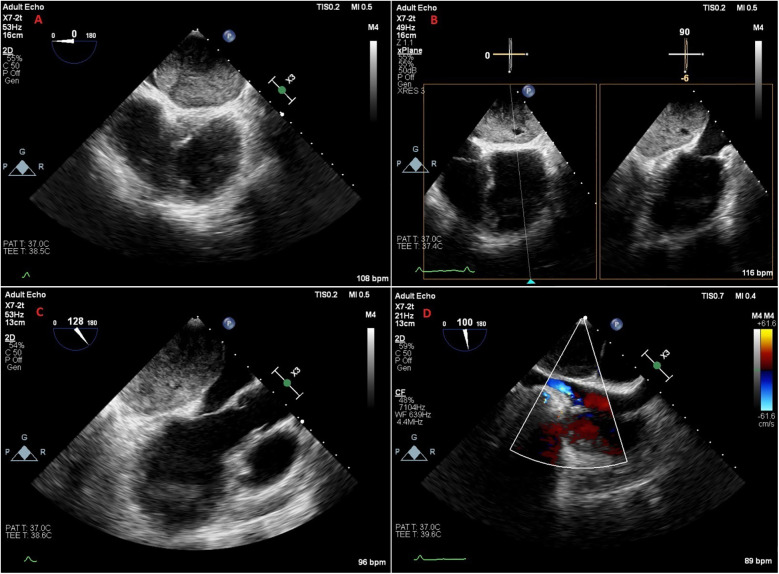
Transesophageal echocardiography images of the patient. (A) Midesophageal 4-chamber view. (B) Biplane view showing that the mass contains an area of cystic degeneration. (C) Midesophageal long axis view showing that the mass is compressing the left atrium, mitral annulus and partially the left ventricle. (D) Midesophageal bicaval view showing the mass creating mild compression on the inferior vena cava.
